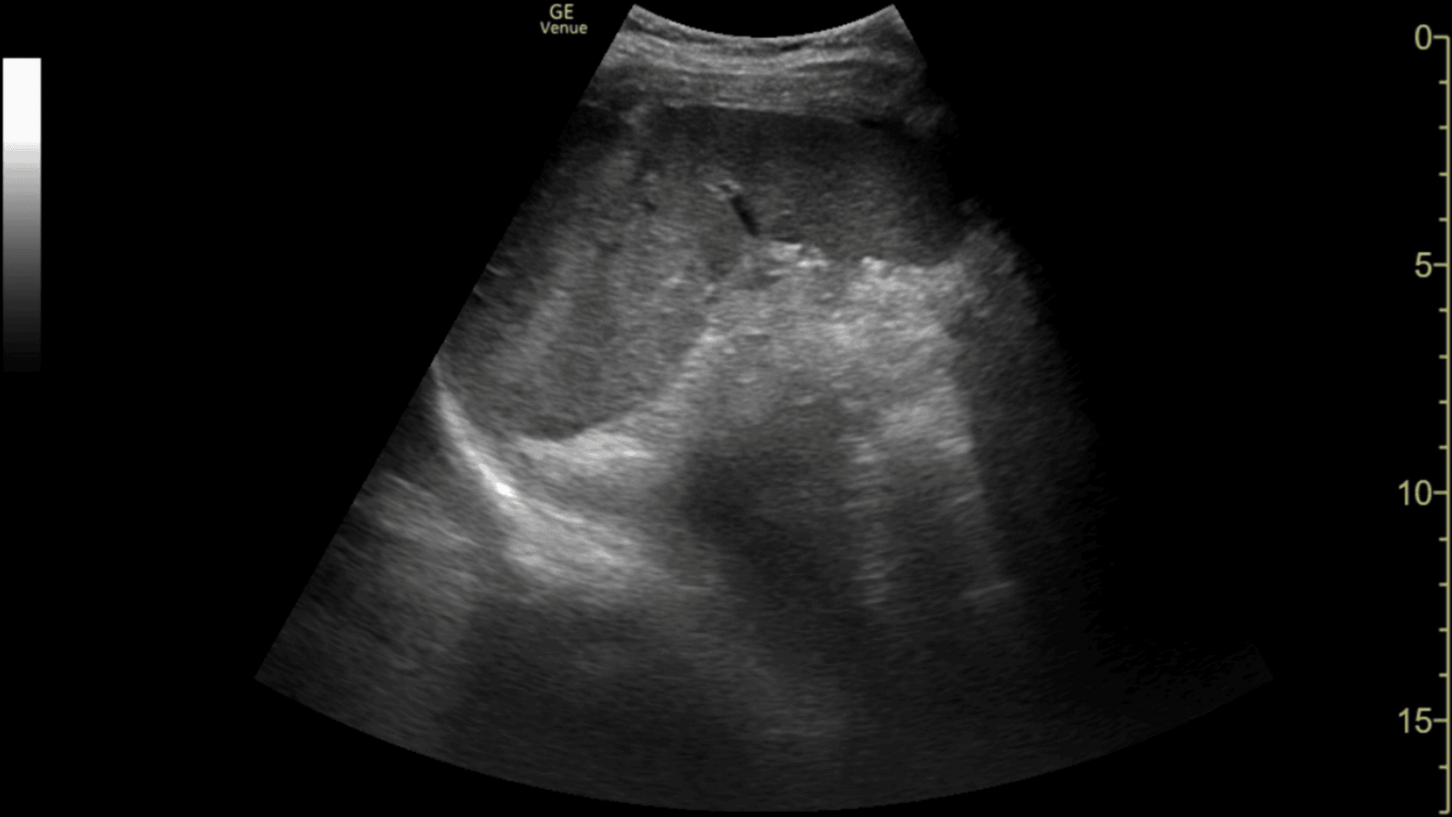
FAST scan of the left upper quadrant showing a normal appearance with no free fluid between the spleen and left kidney FAST: focused abdominal sonography in trauma
